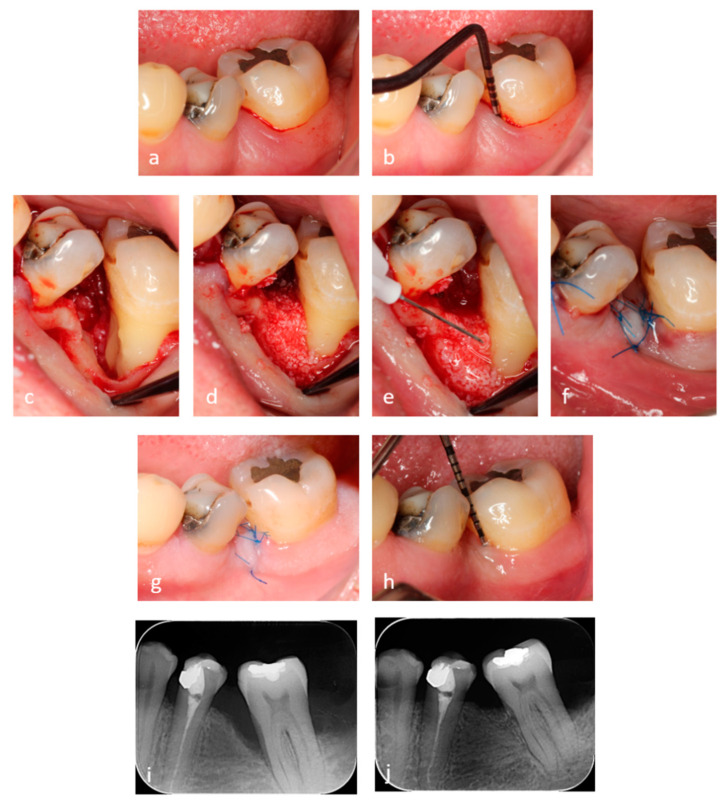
(a,b) Preoperative view and a pocket probing depth (PPD) of 9 mm. (c) Clinical view of the 1-wall intrabony defect and the instrumented root surface with a thin layer of hyaluronic acid (HA) applied. (d) Mixture of HA and xenograft gently packed in the intrabony defect. (e) HA layer applied before suturing. (f) Passive primary wound closure. (g) 14 days healing before suture removal. (h) PPD of 3 mm at 6 months. (i) Pre-operative radiographic finding. (j) Radiographic finding at 6 months.
